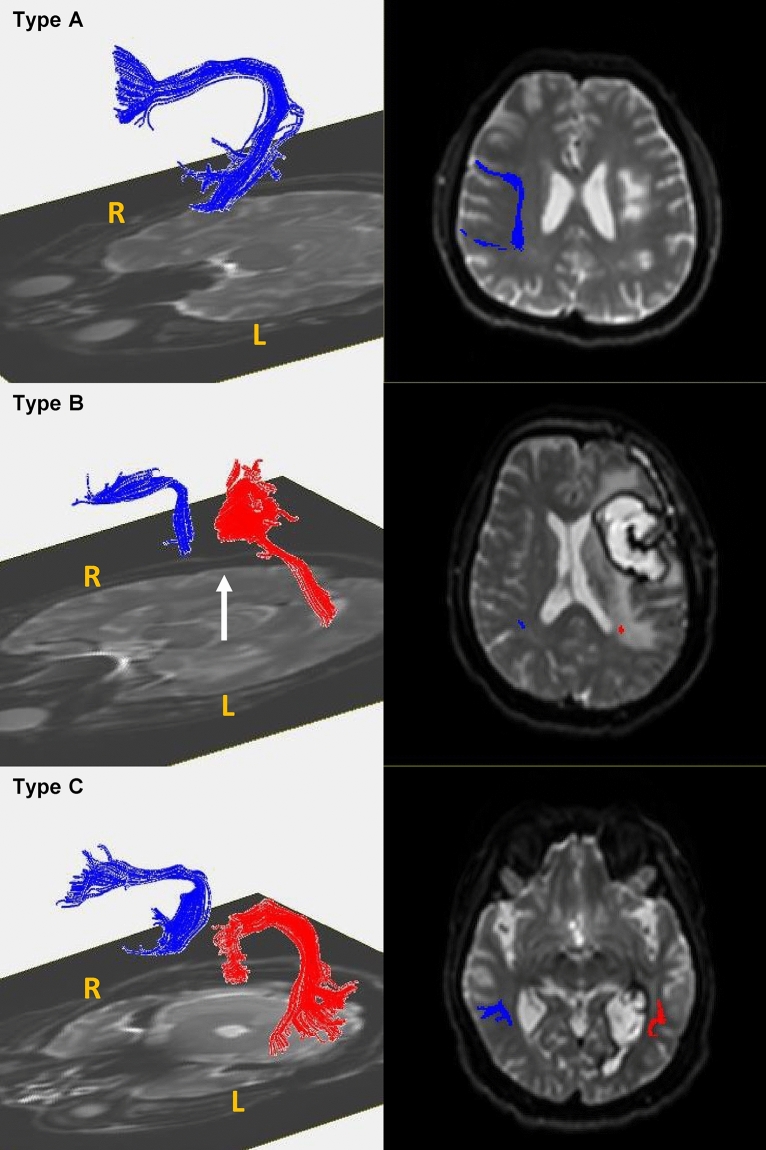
Three types of AF (A–C) displayed according to the severity of left AF damage. (1) Diffusion tensor image of the AF and (2) T2-weighted magnetic resonance image showing three types of AF (A–C; right = blue; left = red) according to the severity of the left AF damage. The white arrow indicates disruption of the left AF surrounding the stroke lesion. *AF* arcuate fasciculus, *R* right, *L* left.
